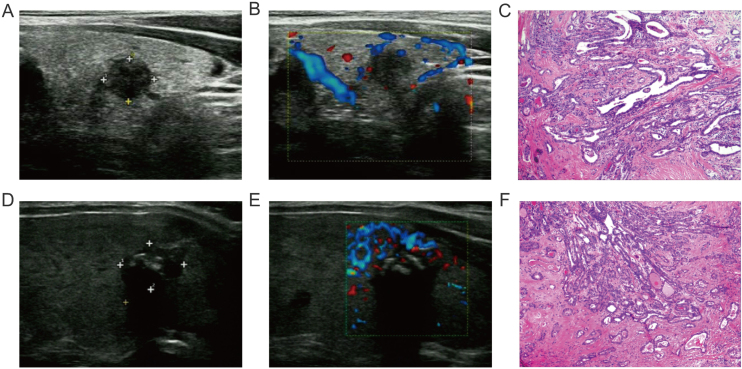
(A‒C) Conventional ultrasonography, CDFI and pathological tissue image of PTC without coexistent Hashimoto’s thyroiditis. (D‒F) Conventional ultrasonography, CDFI and pathological tissue image of PTC with coexistent Hashimoto’s thyroiditis.
